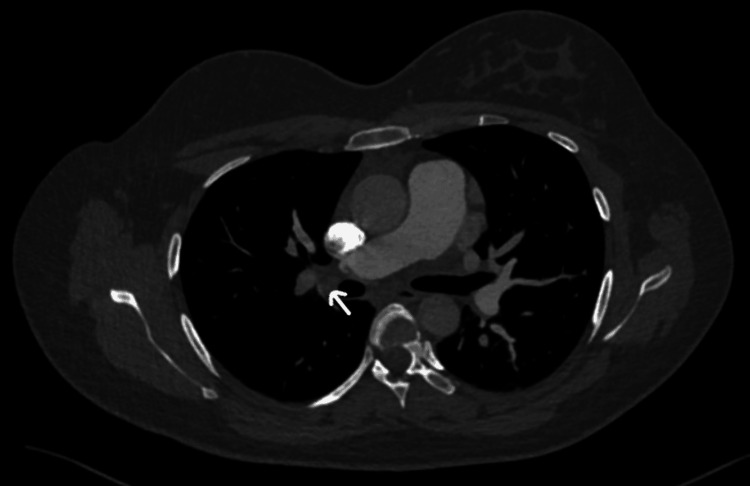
CT pulmonary angiogram (right pulmonary artery) There is evidence of filling defects seen in the right pulmonary artery
